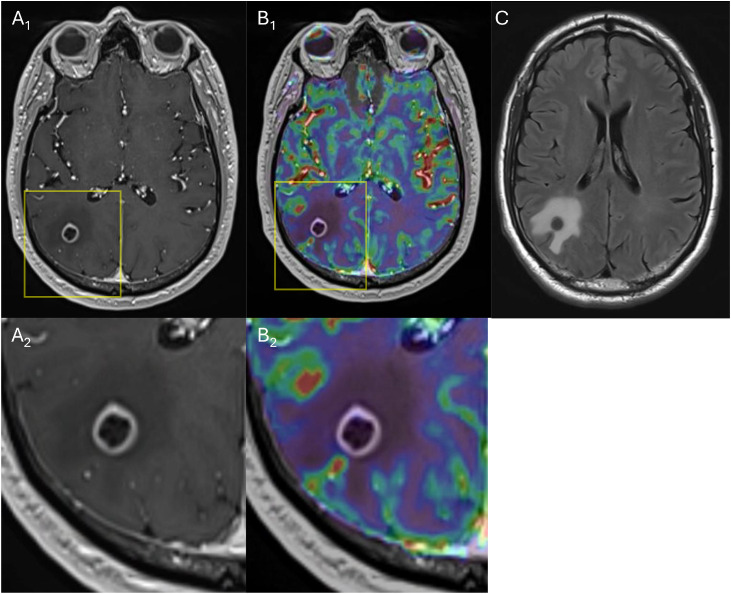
A magnetic resonance imaging (MRI) scan of the brain with dynamic susceptibility contrast (DSC) perfusion performed 8 months after linac-based stereotactic radiosurgery (SRS). (A_1_) Axial T1-weighted postcontrast (T1c+) demonstrates a 1.3 × 1.3 cm peripherally enhancing lesion in the right parietal lobe; the yellow box denotes the magnified view in (A_2_). (B_1_) DSC perfusion overlay demonstrates low perfusion within the enhancing components; the yellow box denotes the magnified view in (B_2_). (C) Axial T2 fluid-attenuated inversion recovery (FLAIR) demonstrates surrounding edema.
